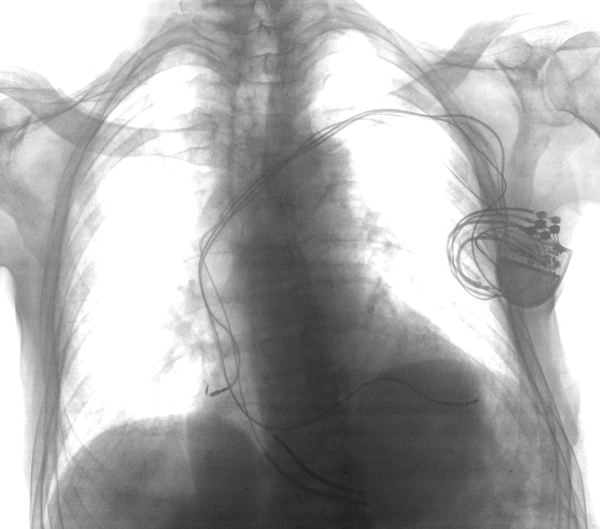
Chest X-ray before the procedure showing two electrocatheters in the right ventricle, one in the coronary sinus and a right auricular catheter.
